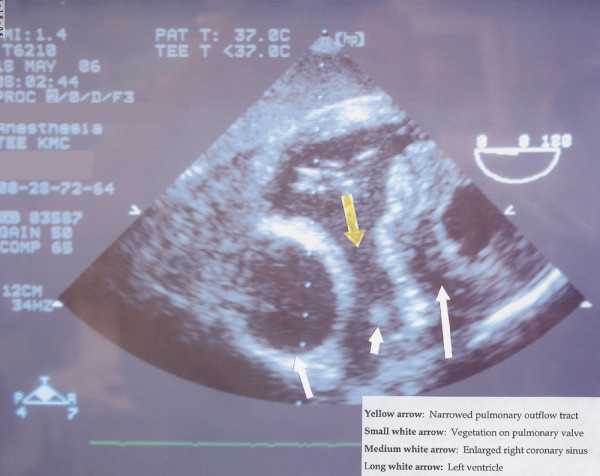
A transesophageal echocardiogram depicting an enlarged right coronary sinus (medium white arrow) and identification of the vegetation on the pulmonary valve (small white arrow).
